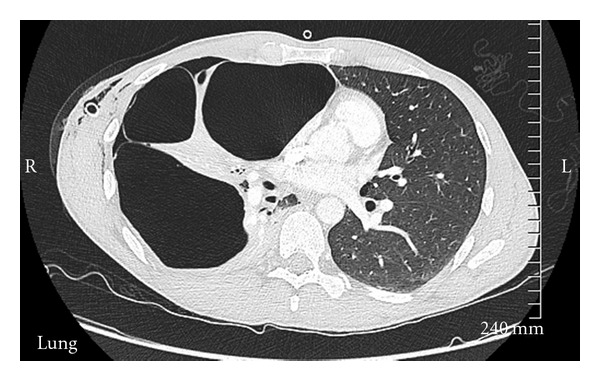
Chest CT at different section showing bullous disease.
